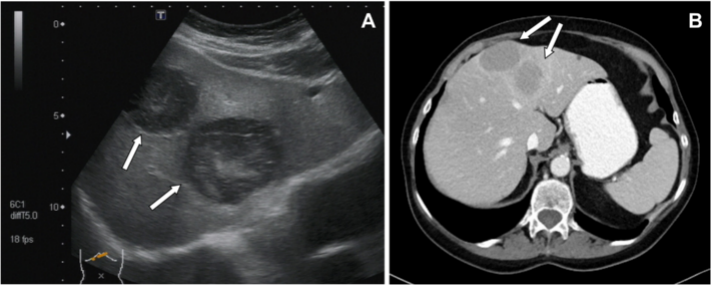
Imaging of the liver lesions (→) with ultrasound (a) and CT (b)
